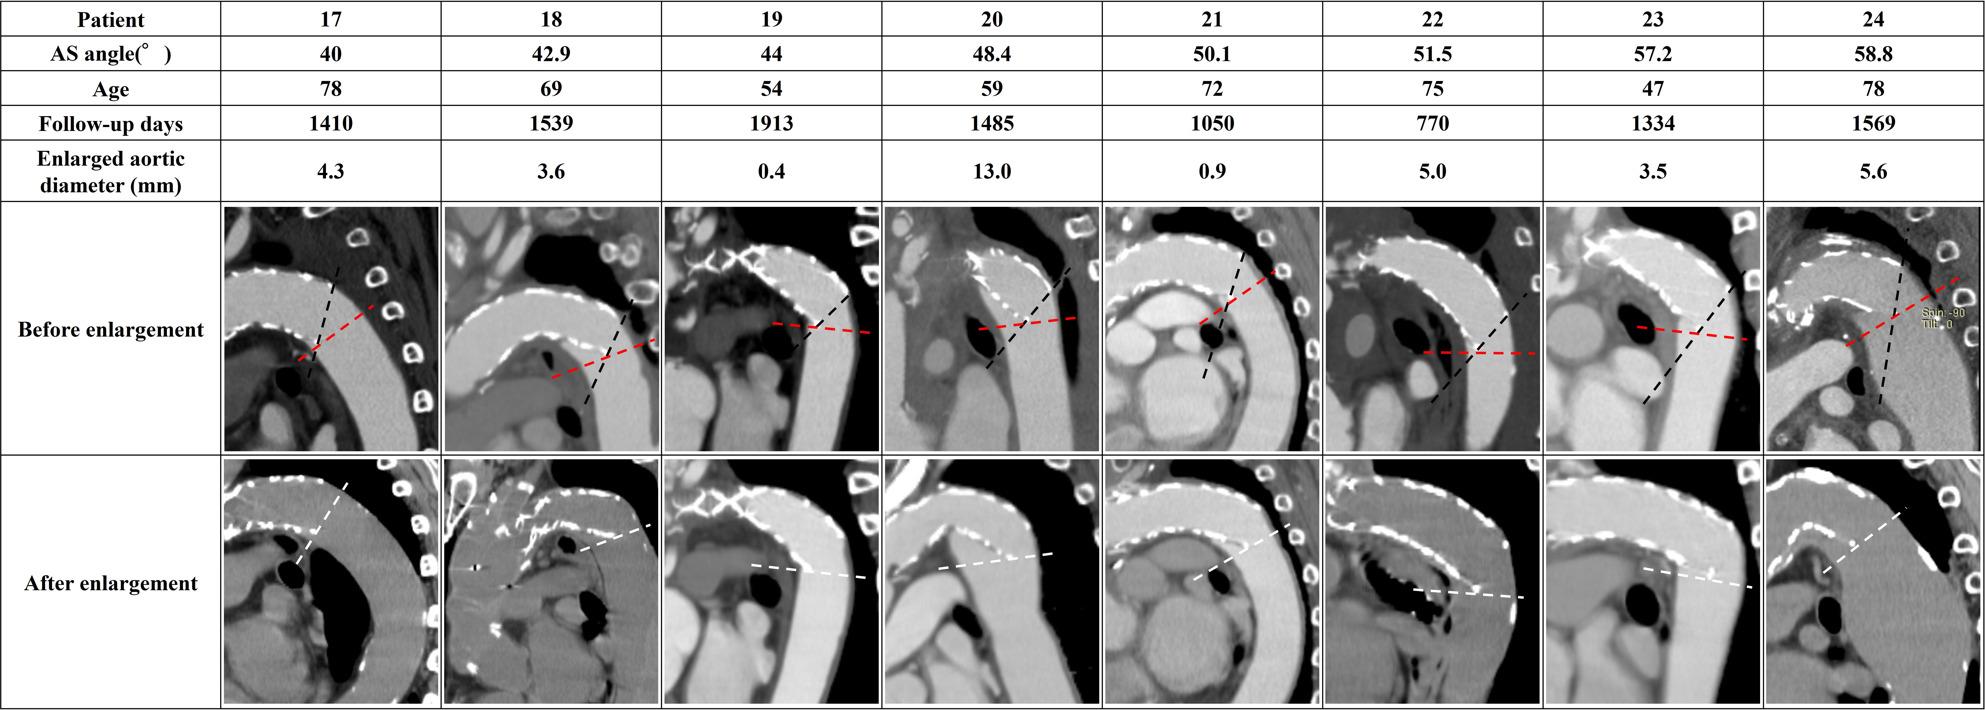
Patient-specific AS angles and CT views (Patients 17–24)
